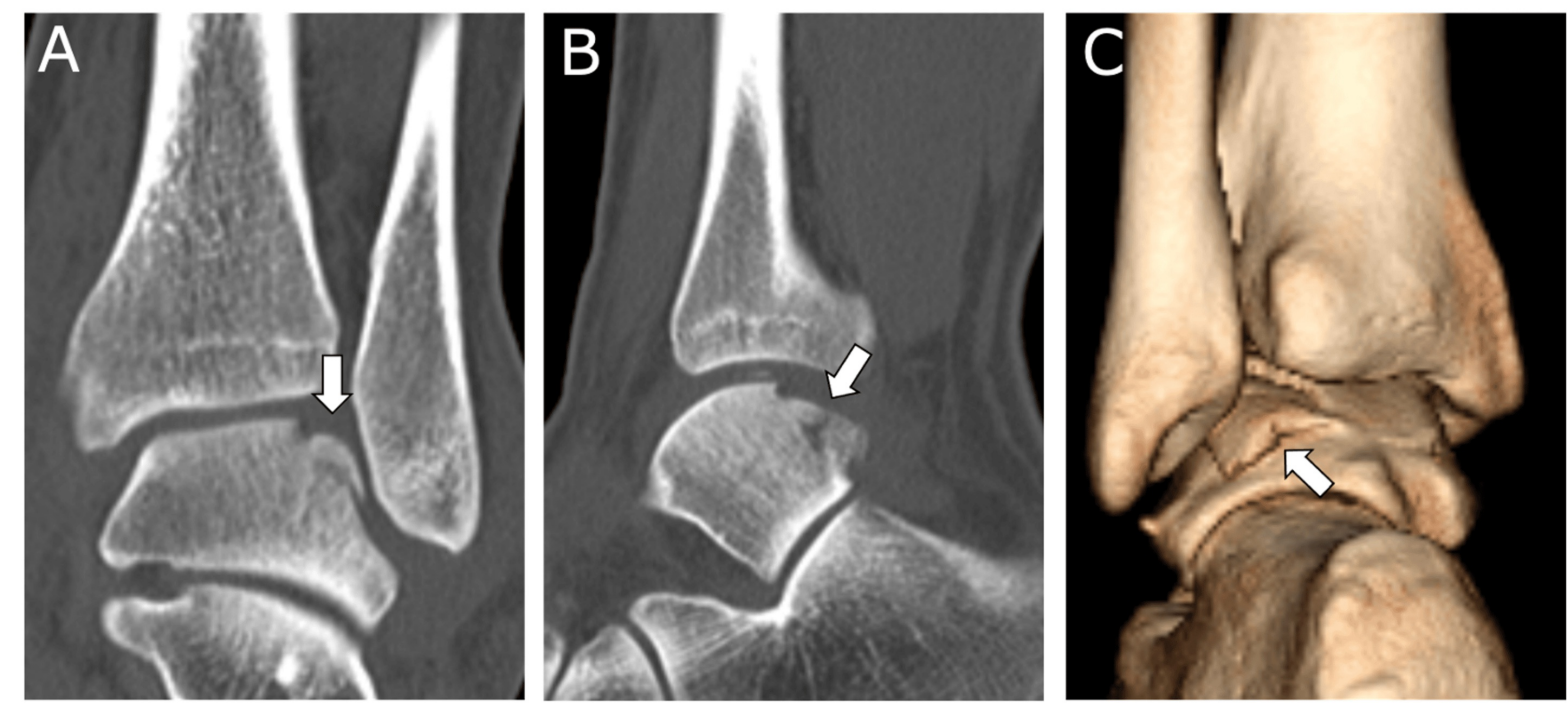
Preoperative plain computed tomography (CT) images A displaced osteochondral lesion is identified in the posterolateral talar dome (arrow). A: Coronal view; B: Sagittal view; C: Three-dimensional CT reconstruction
